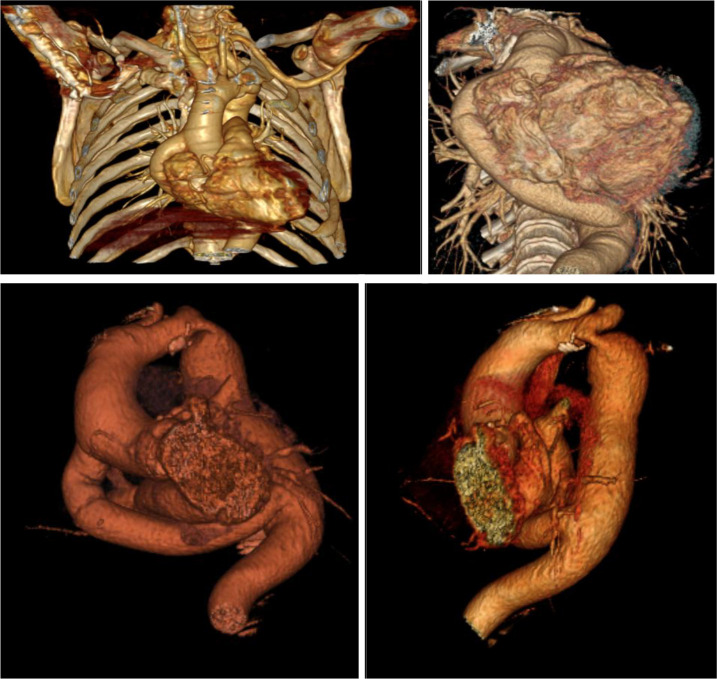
Reconstruction of an angio-computed tomography scan of a
